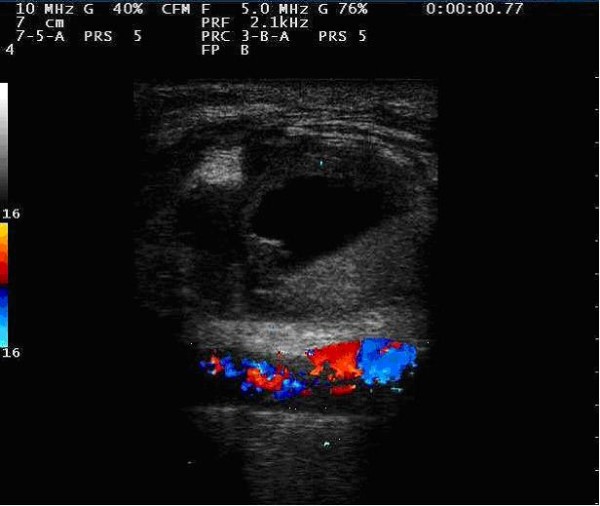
Post-operative Duplex ultrasound control assessing the complete exclusion of the lesion.
